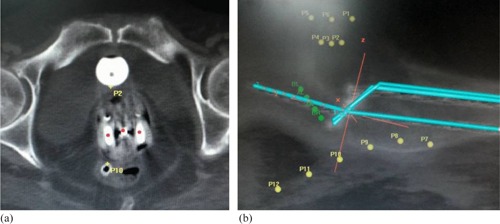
CT Scan images showing the marking of bladder and rectal points: (a) axial CT image showing ICBT applicator with central tandem and two ovoids where the bladder point can be seen on the posterior surface of Foley's bulb and the rectal point can be seen on the anterior surface of marker tube inserted into the rectum; (b) sagittal CT image showing the reconstructed ICBT applicator, multiple bladder points anteriorly and rectal points posteriorly.
